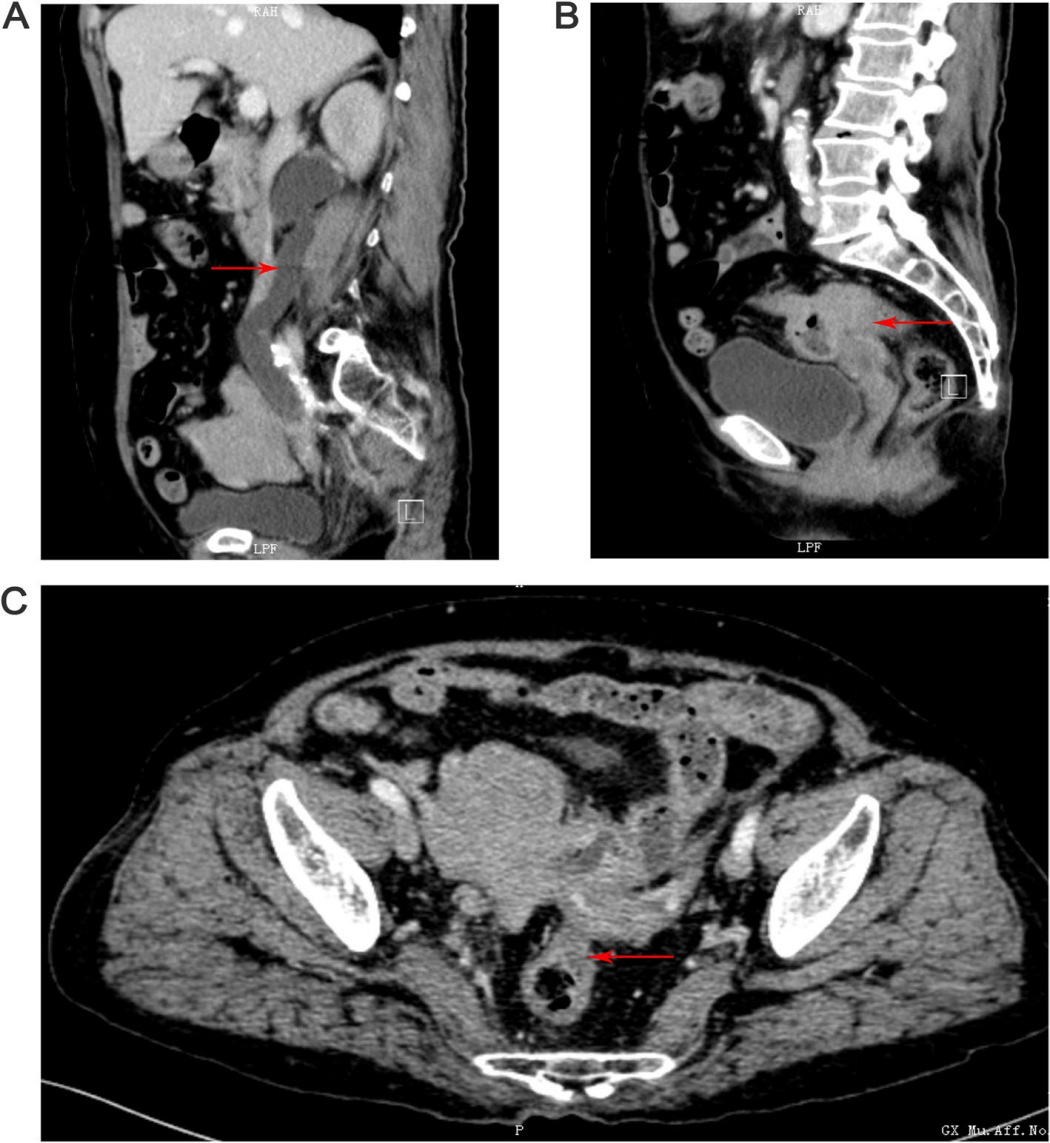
The computed tomography scan of pelvic cavity. A The ureterectasis due to compression of large masses in the lower part of the right-side ureter. B The upper rectal mass and uterine adhesion were not clearly demarcated. C The thickening of the rectal wall and the adhesion of the adnexa are not clearly demarcated. (see red arrows)
